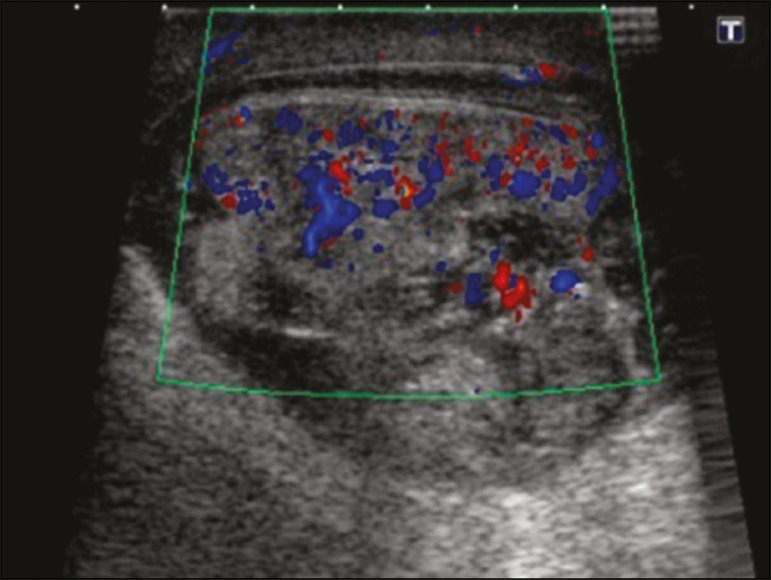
Ultrasound of the same patient depicted in Figure 14, showing a contiguous, heterogeneous fluid collection in the left scrotum, together with fluid collections in the pancreas and groin.
